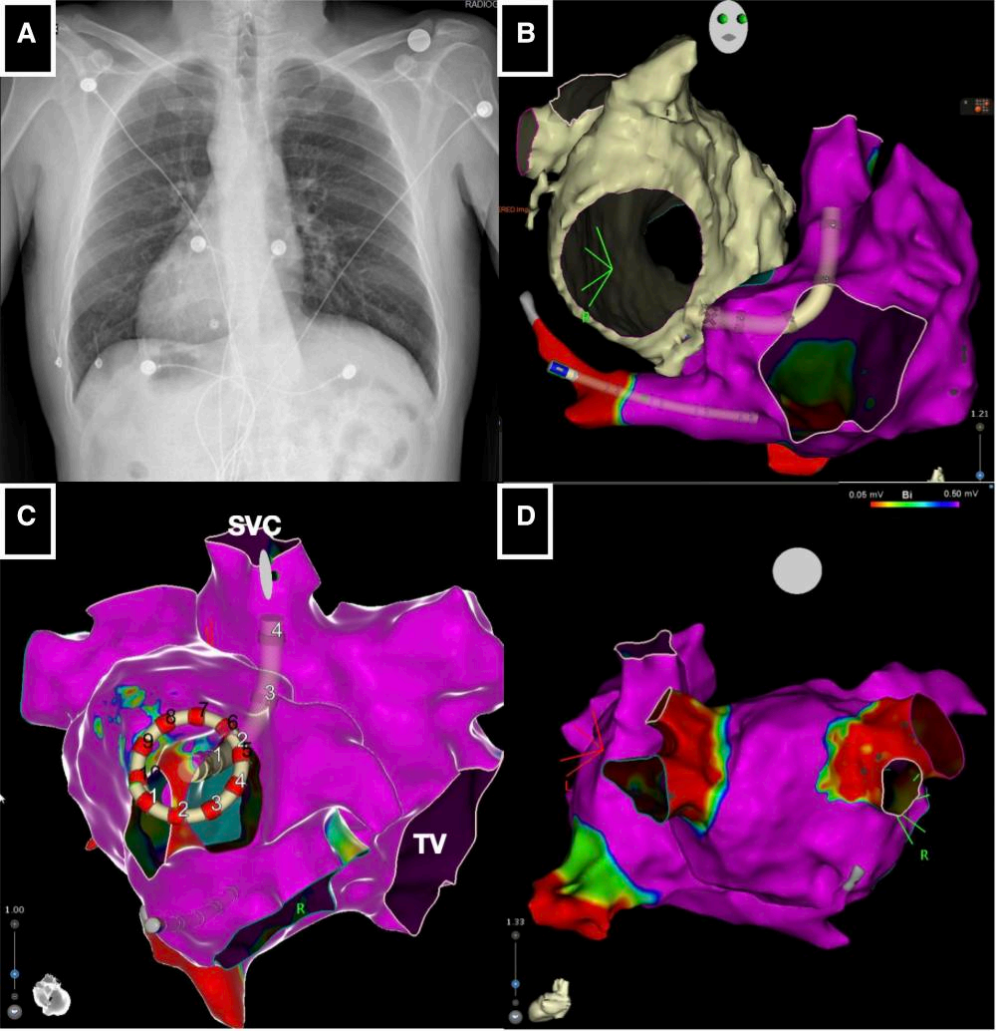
Pulsed field ablation of atrial fibrillation in a patient with situs viscerum inversus, dextrocardia, and interrupted inferior vena. (*A*) Standard chest radiography highlighting dextrocardia. (*B*) Electroanatomical mapping (RAO projection mimicking LAO) with cardiac magnetic resonance integration guiding the positioning of the VIZIGO sheath at the fossa ovalis. (*C*) Electroanatomical mapping guiding Varipulse catheter positioning within anatomical right pulmonary veins. (*D*) Electroanatomical mapping post-ablation confirming complete pulmonary vein isolation with PFA.
